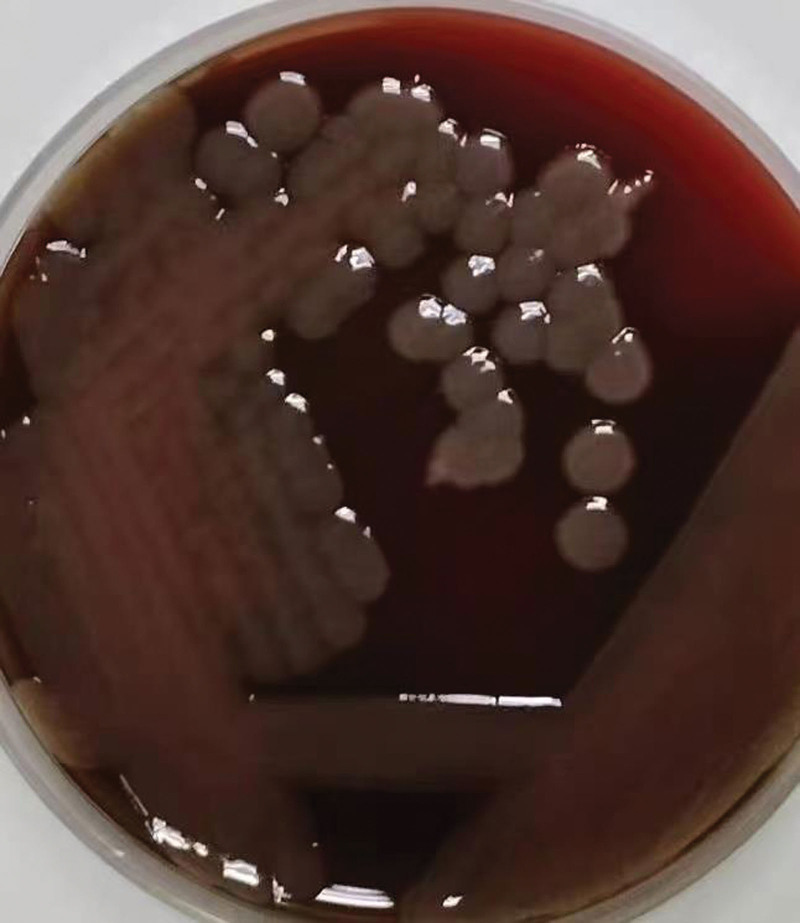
Chest computed tomography results of the patient after admission.
